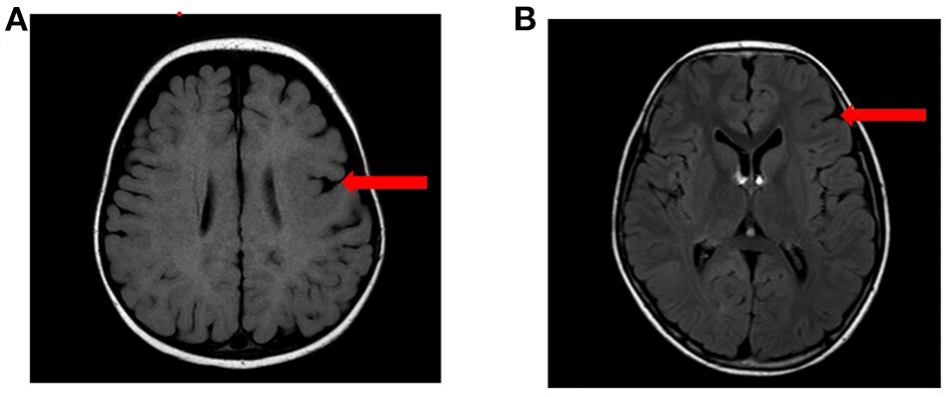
The MRI results of the patients. (A) MRI results of case 3: left frontal MCD. (B) MRI results of case 10: left frontal FCD.
